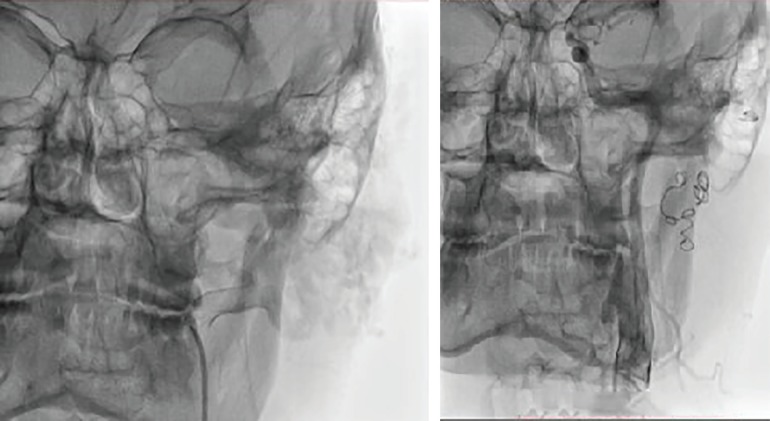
MRI and MR Angiography showing feeding vessel arising from the left external carotid artery
